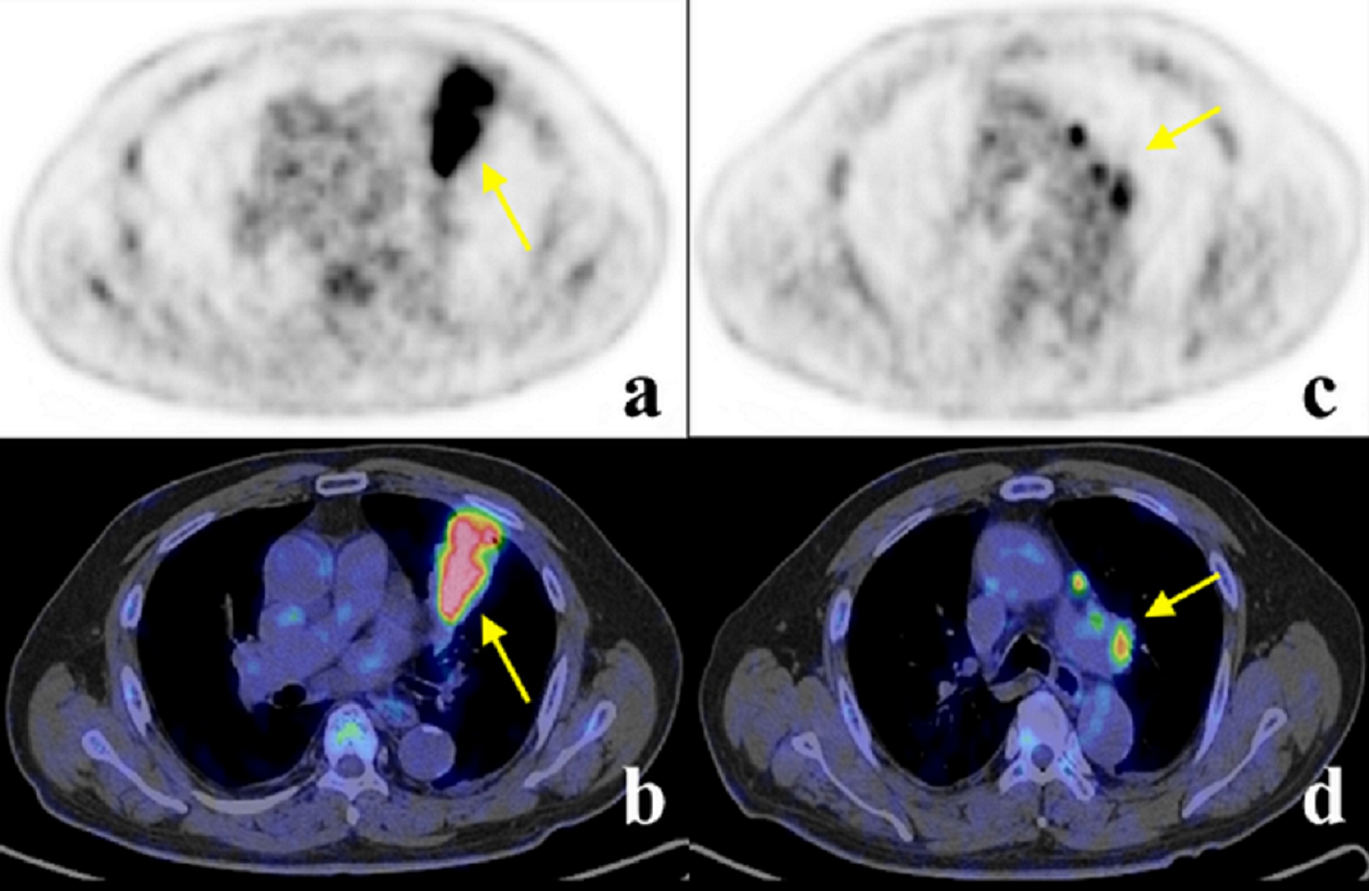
The F-18-FDG PET-CT The F-18-FDG PET-CT showed a high uptake around the mass and the lymph nodes. (a) Tumor level PET; (b) tumor level PET-CT; (c) lymph nodes level PET; (d) lymph nodes level PET-CT. F-18-FDG: fluorine-18 fluorodeoxyglucose
